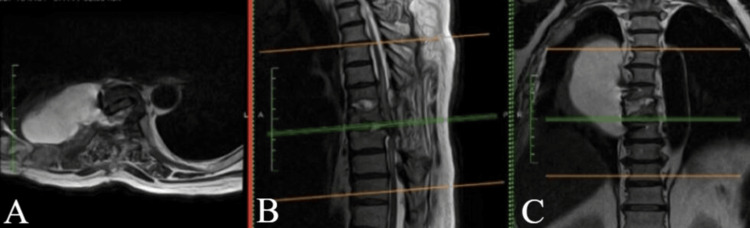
T2-weighted MRI, identifying cerebrospinal fluid fistula likely due to a T7-T8 defect in the axial (A), sagittal (B), and coronal (C) plane.
